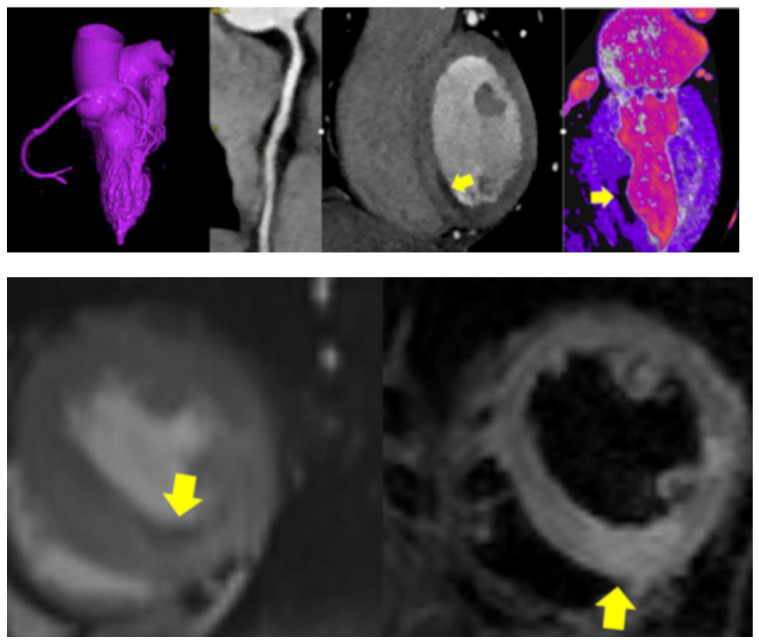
CTA showed diffuse vessel wall irregularities with hazy wall thickening of the RCA (upper panel, left), and signs of perivascular inflammation (PCAT −44 HU). Both CTA (upper panel) and MRI (lower panel) showed midventricular inferior perfusion defects (hypodense, yellow arrow) (left) and myocardial edema (right) inferior on T2 MRI sequences (yellow arrows). (upper panel): coronary computed tomography angiography (CTA) and (lower panel): magnetic resonance imaging (MRI) with perfusion sequence (left) and T2 (right). A final diagnosis of MINOCA was made.
